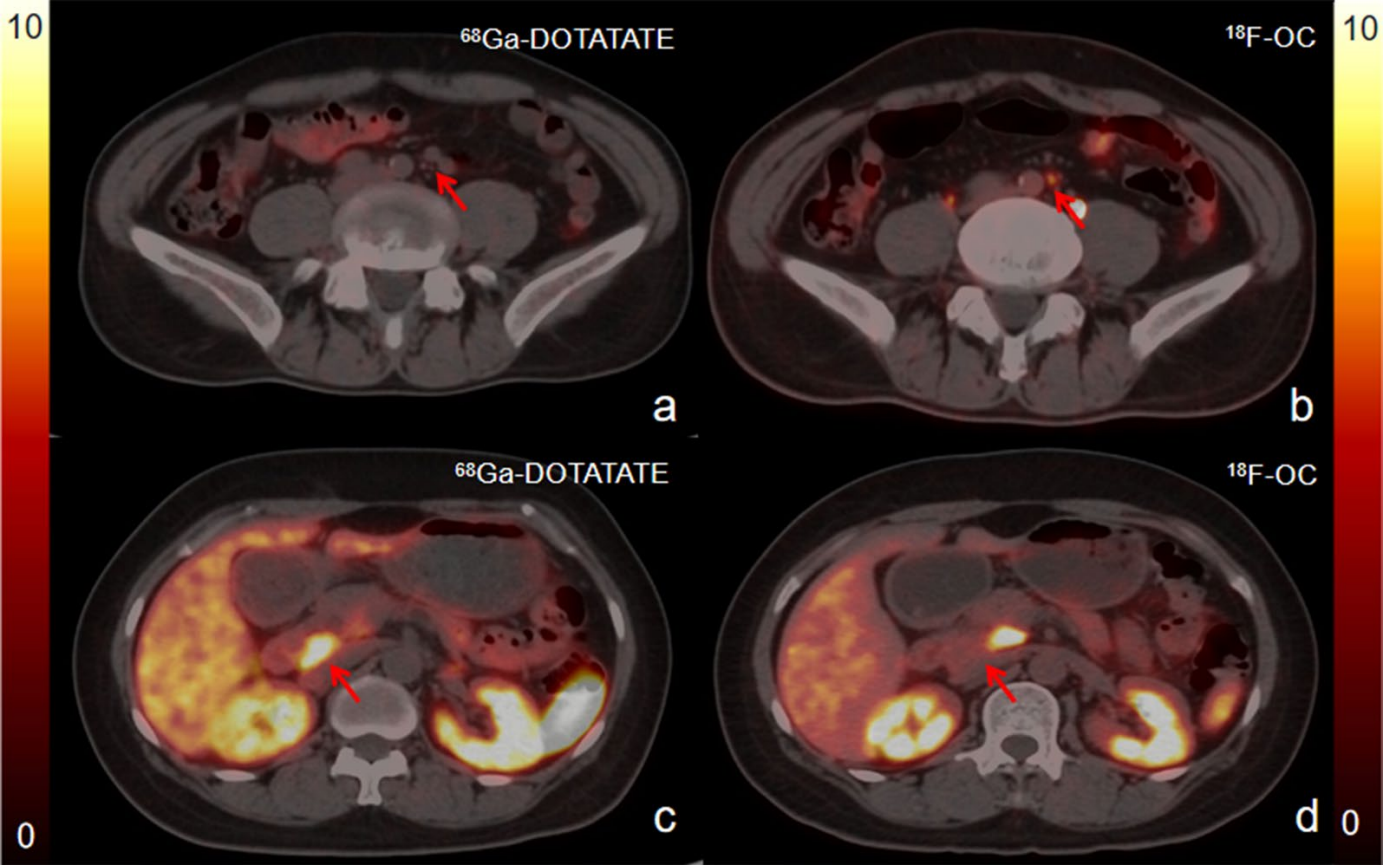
Both ^18^F-OC and ^68^Ga-DOTATATE had lesions that could not be detected by another imaging agent. PET/CT images acquired with ^68^Ga-DOTATATE and ^18^F-OC for patient No. 19 (a, b) and No. 20 (c, d). The ^18^F-OC fusion image (b) found an increased uptake in a retroperitoneal lymph node, while the uptake of this lymph node was not significantly increased on ^68^Ga-DOTATATE (a) image. However, in another patient, the ^18^F-OC fusion image (d) shows that the uptake in one retroperitoneal lesion was not obvious, while the uptake of ^68^Ga-DOTATAE was significant (c)
